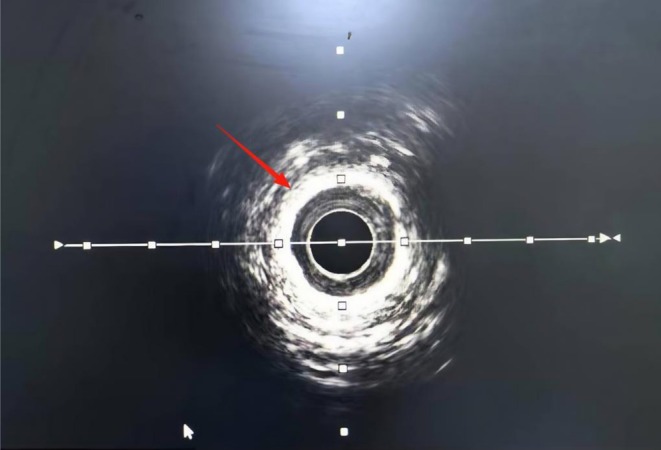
360° circumferential coronary calcification observed using intravascular ultrasound. The bright, continuous echogenic ring encircling the lumen reflects extensive calcific plaque, indicating a rigid vessel wall before treatment.
